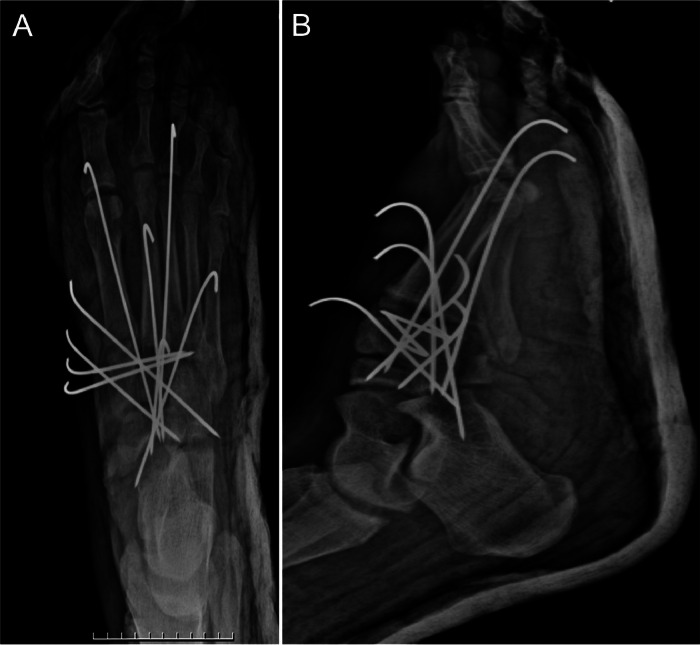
Follow-up plain radiographs of the right foot taken 2 weeks postoperation. (A) Anteroposterior (AP) view, (B) Lateral view. The medial cuneiform and metatarsals remain in normal alignment.
